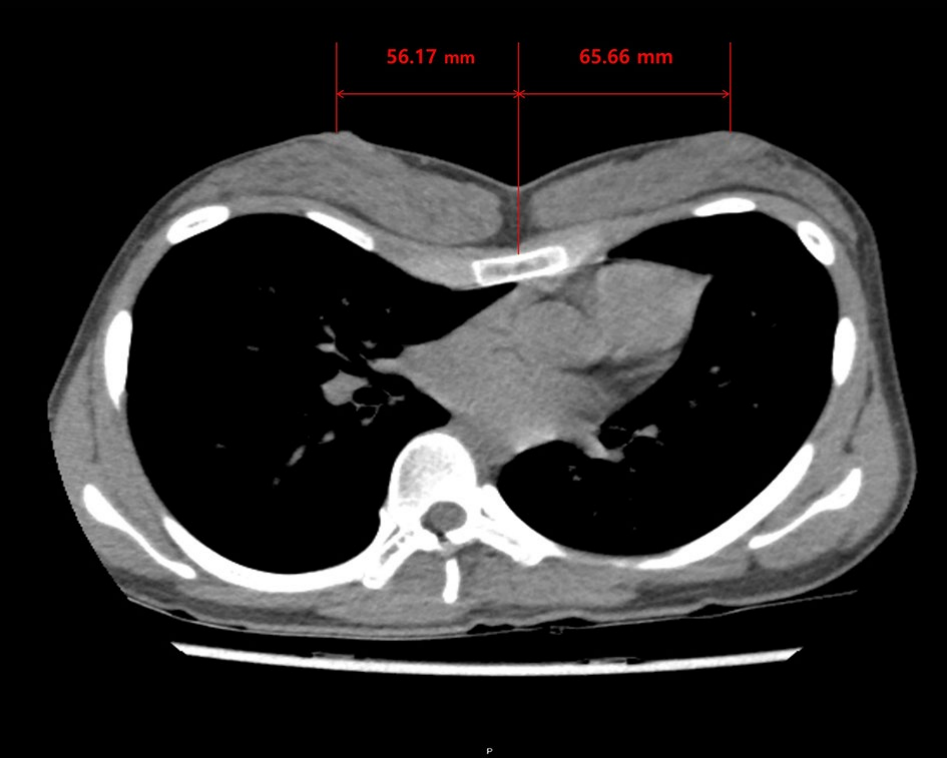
Measuring the distance between the nipples. Each distance from the sternal midline to the right and left nipples based on chest computerized tomography (CT) was measured and the distance between the nipples was calculated as the sum of the right and left distances.
